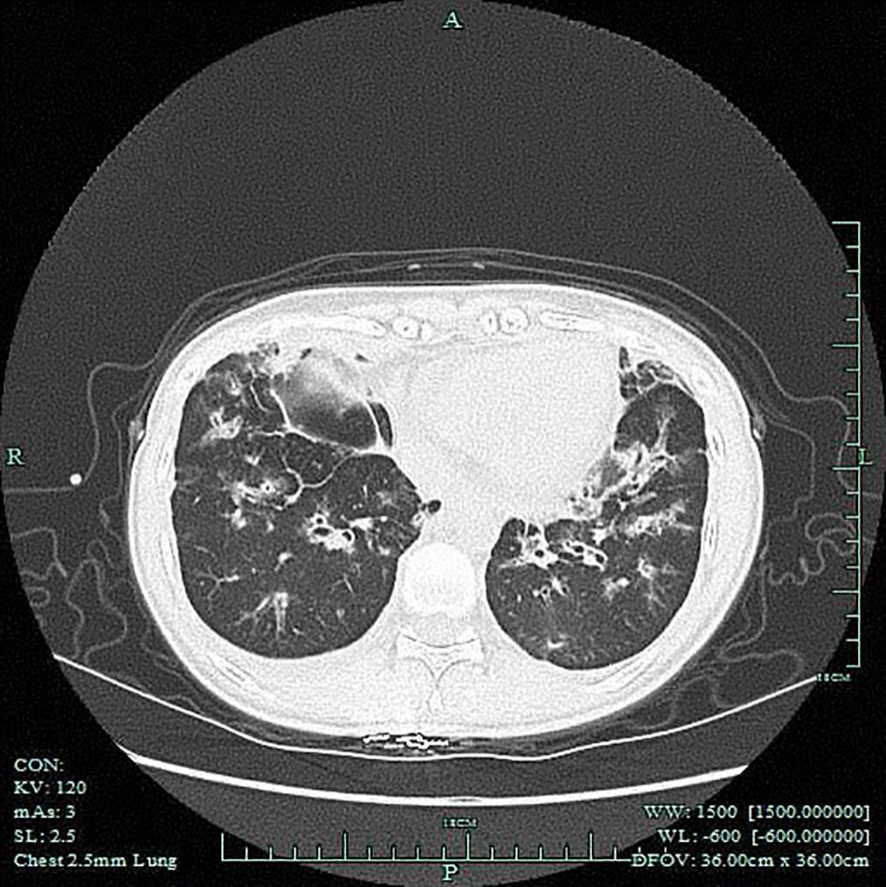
Chest CT scan on the day of admission. Images demonstrate bronchiectasis accompanied by extensive exudative opacities in both lungs, consistent with pulmonary involvement of EGPA.
